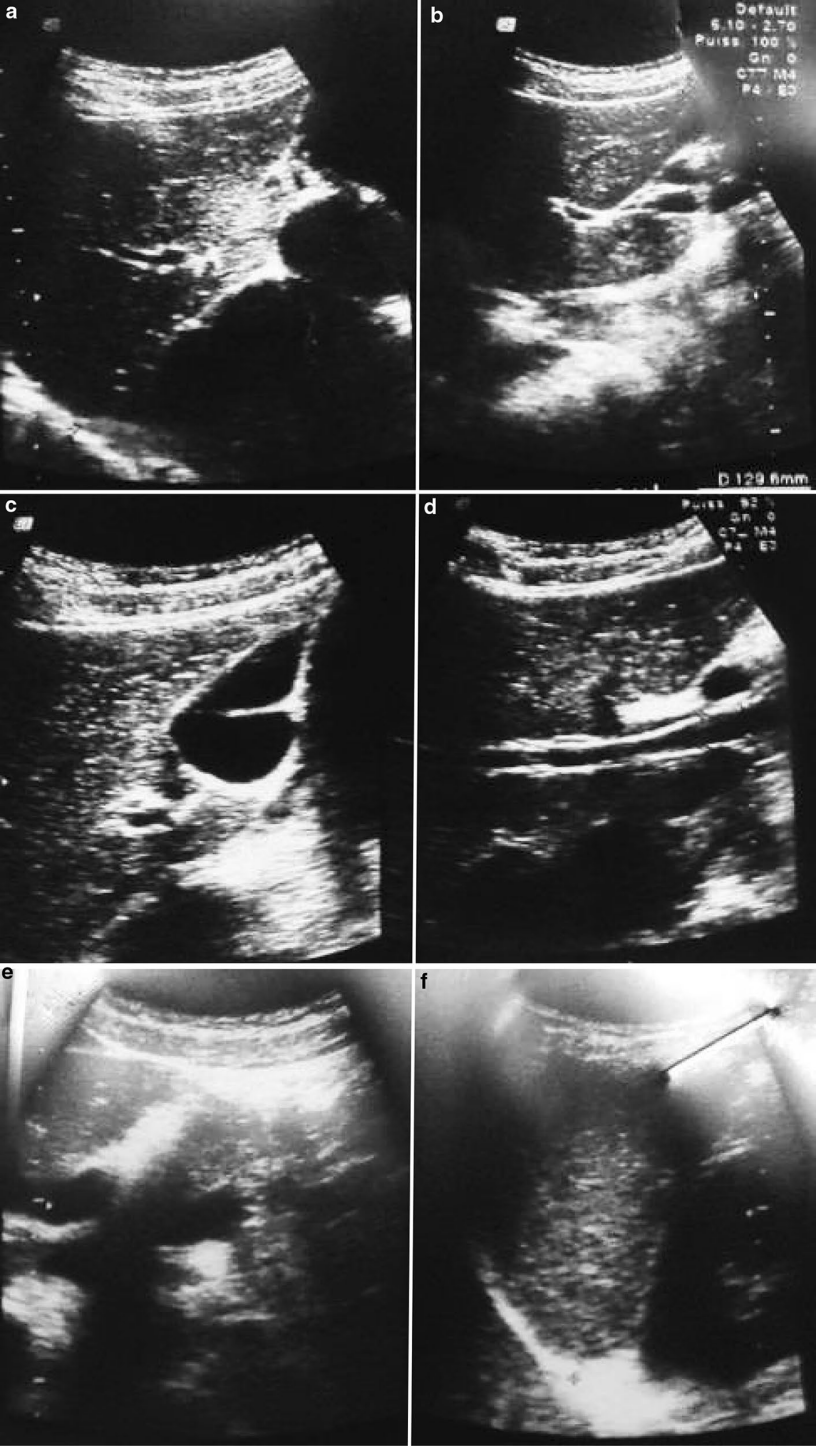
Abdominal ultrasound showing the other abdominal viscera. The right (a) and left (b) liver lobes, the gall bladder (c), pancreatic duct (d), the pancreas (e) and spleen (f) are all normal. No viscus anomaly detected
